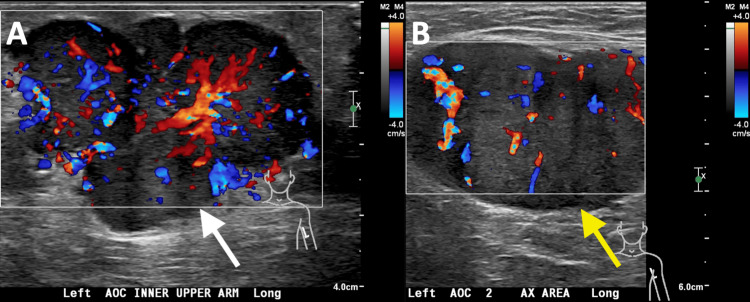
A. Targeted ultrasound of the left upper arm with color Doppler demonstrating a circumscribed, hypoechoic, polypoid mass (white arrow) with increased internal vascularity. B. Targeted ultrasound of the left axilla revealing a well-circumscribed, hypoechoic oval mass (yellow arrow) with increased internal vascularity.
